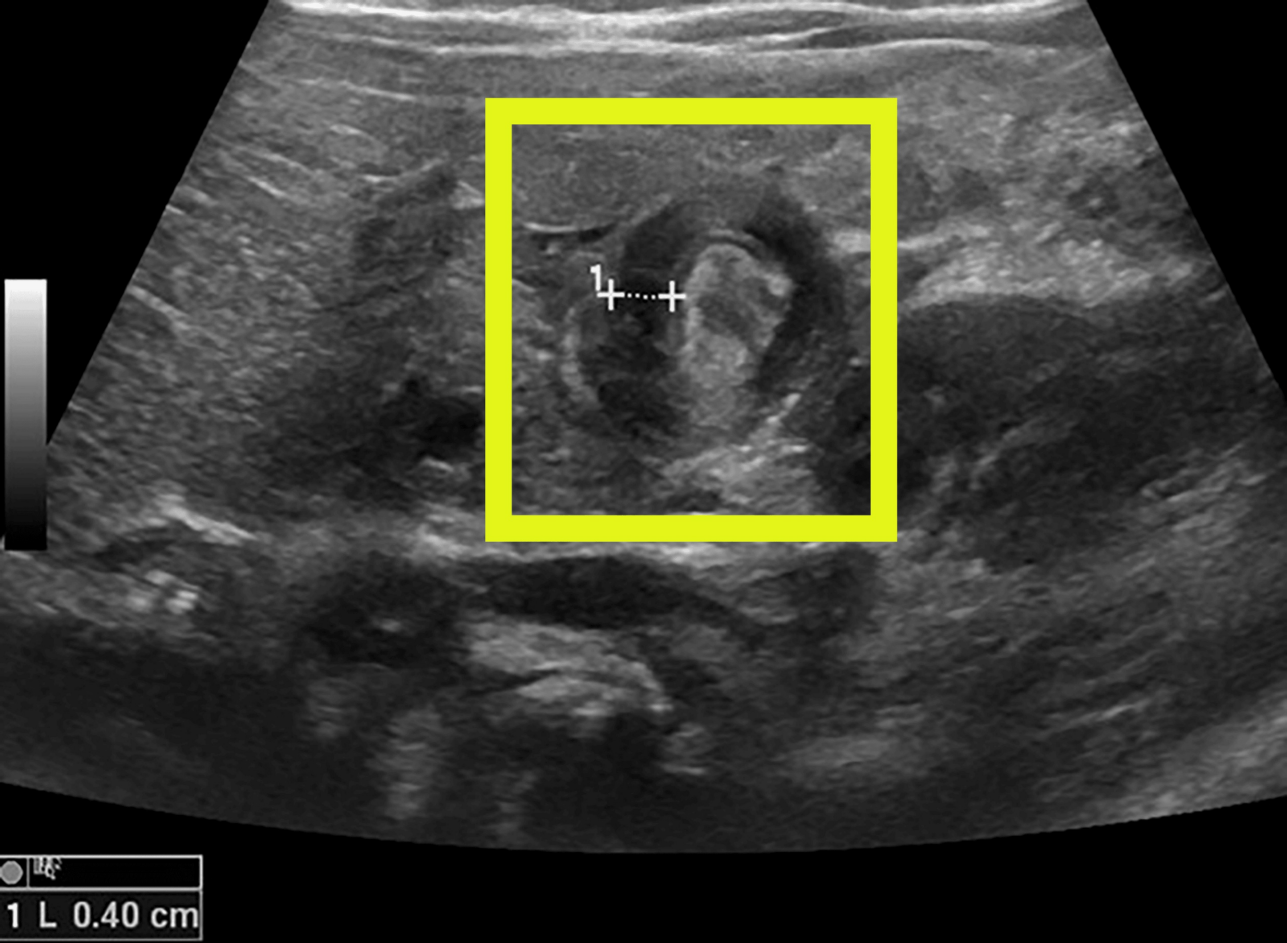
Transverse ultrasonography through the pylorus (yellow box) shows a thickened hypoechoic pyloric muscular wall and central echogenic mucosa, a typical “doughnut” appearance in IHPS. IHPS, Infantile Hypertrophic Pyloric Stenosis
